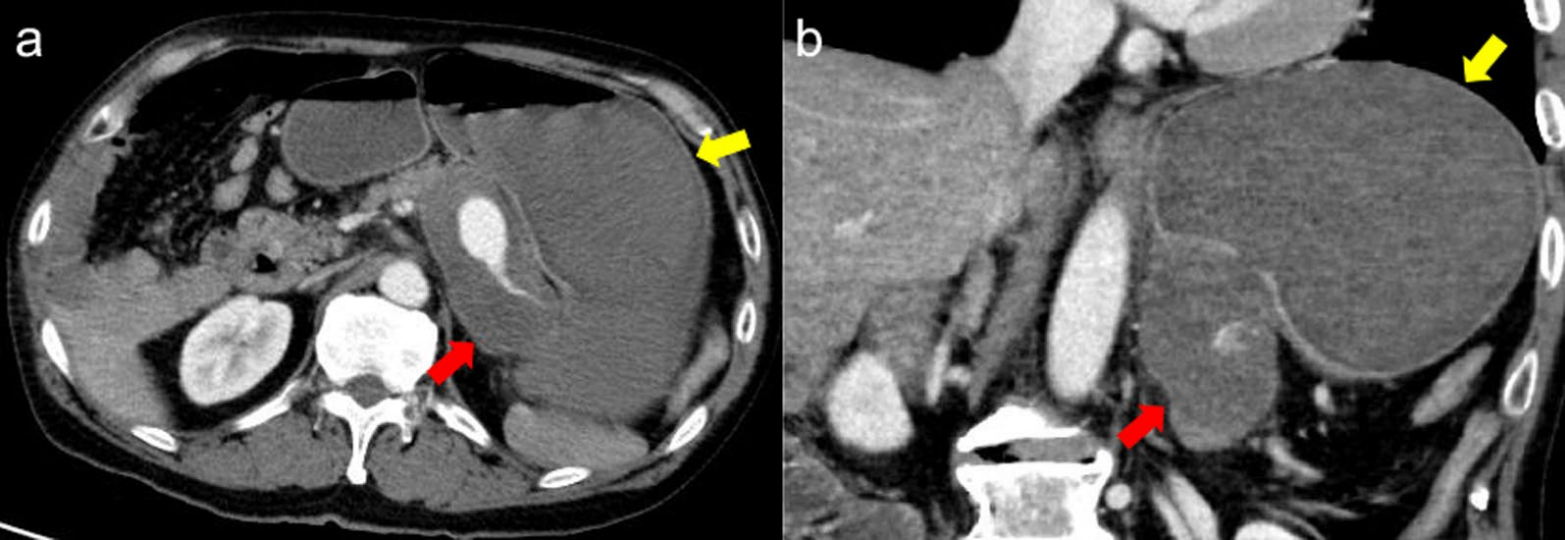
Abdominal contrast-enhanced computed tomography findings. a Splenic artery aneurysm (SAA) (10 cm in maximum diameter) with extravasation of contrast from the splenic artery. The red arrow indicates the SAA, and the yellow arrow indicates the stomach. b SAA in close communication with the posterior wall of the stomach. The red arrow indicates the SAA, and the yellow arrow indicates the stomach
